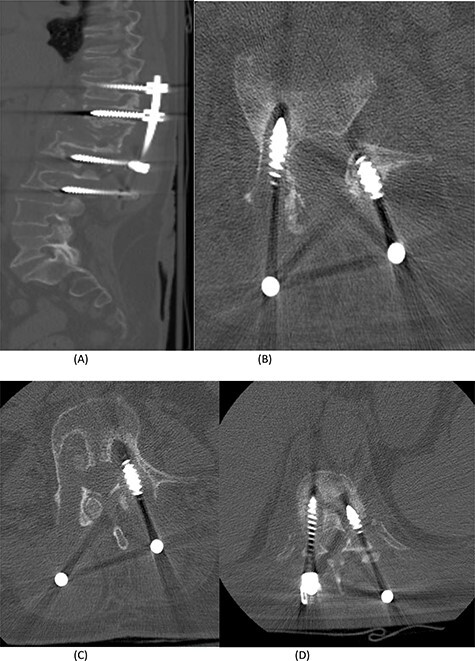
(A) Sagittal spine CT demonstrating a burst fracture involving L1 vertebral body with retropulsion of fragments and epidural soft tissue mass indenting the conus medullaris at the level of T12–L1. (B) Axial spine CT of the corresponding level of L2 vertebral body. (C) Axial spine CT of the corresponding level of L3 vertebral body. (D) Axial spine CT of the corresponding level of T11 vertebral body.
